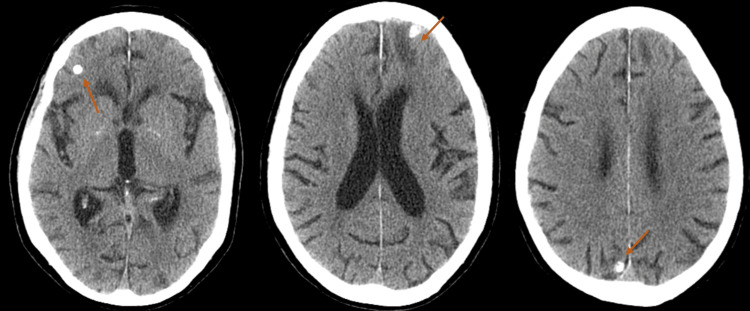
Computed tomography images with calcified lesions, the middle lesion with perilesional oedema
